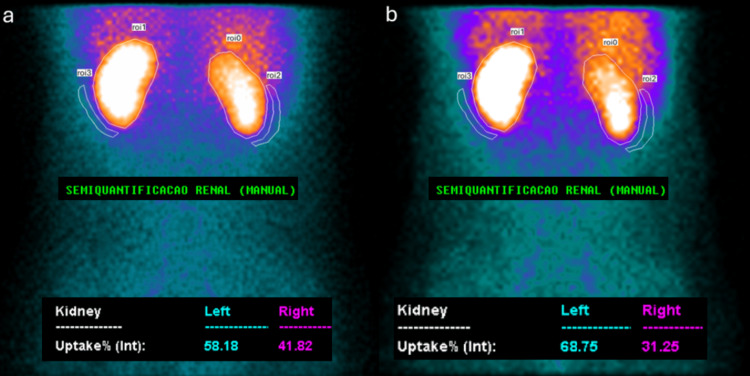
(a) Baseline 99mTc-DTPA renal scintigraphy demonstrating a semi-quantitative differential renal function of 42% for the right kidney and 58% for the left kidney. (b) Captopril-enhanced 99mTc-DTPA renal scintigraphy showing a semi-quantitative differential renal function of 31% for the right kidney and 69% for the left kidney.
